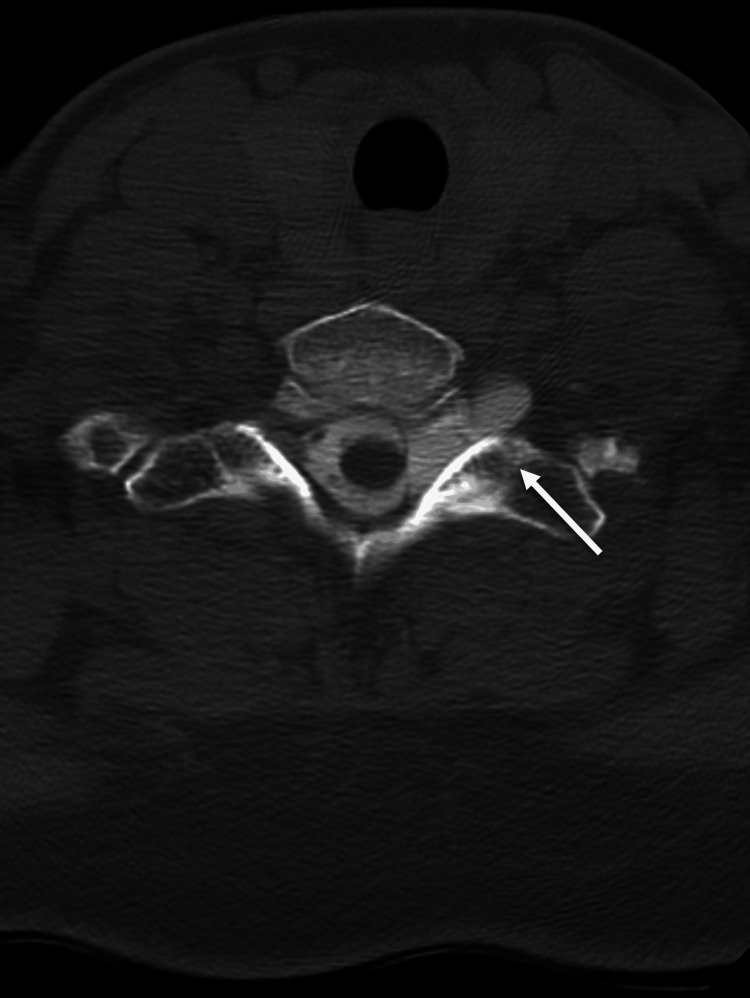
Delayed computed tomography myelogram showing pseudomeningocele typical of root avulsion (arrow).
